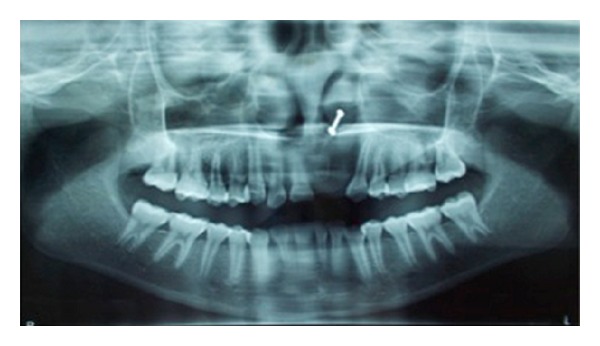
Orthopantomograph showing missing tooth number 21 and tooth number 22.
